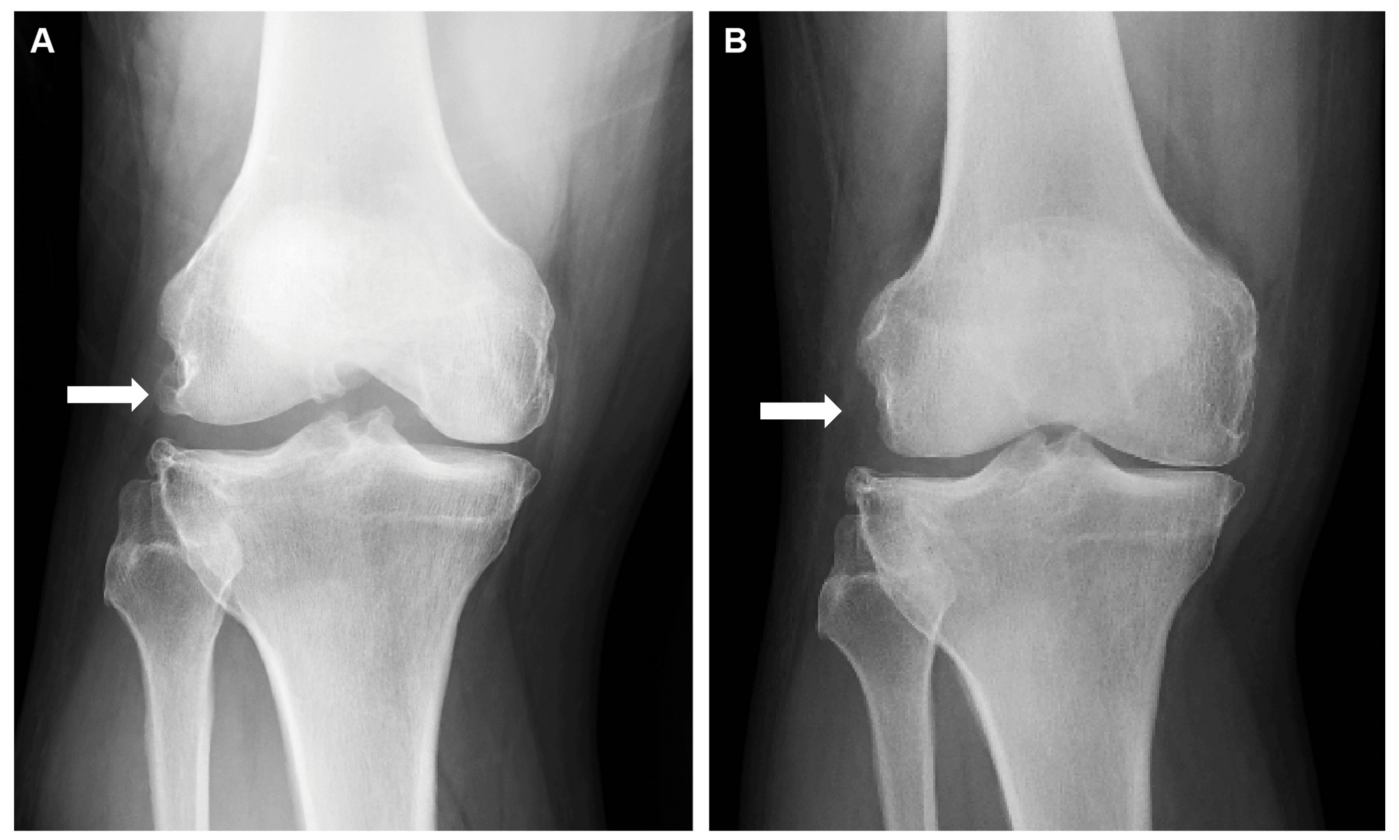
Preoperative (A) and postoperative (B) X-ray images of the right knee. The arrow in the preoperative X-ray shows a large osteophyte on the lateral femoral condyle that impinges on the popliteal tendon. The postoperative X-ray confirms the absence of an osteophyte following arthroscopic resection as indicated by the arrow. LFC: lateral femoral condyle
